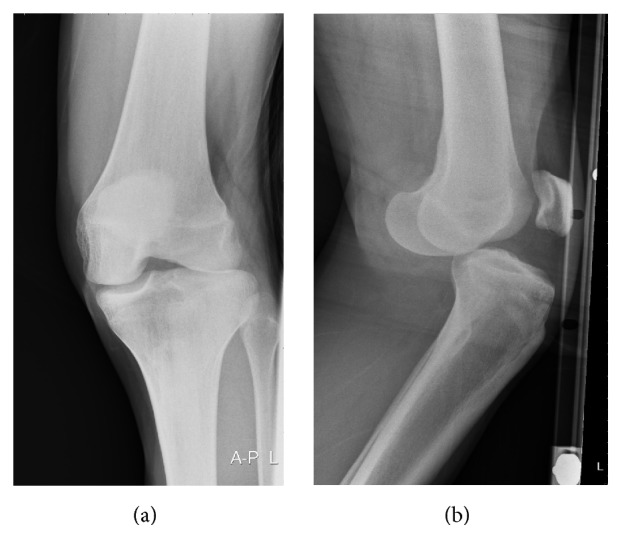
Anteroposterior and lateral radiographs showing anterolateral dislocation of the left knee.
